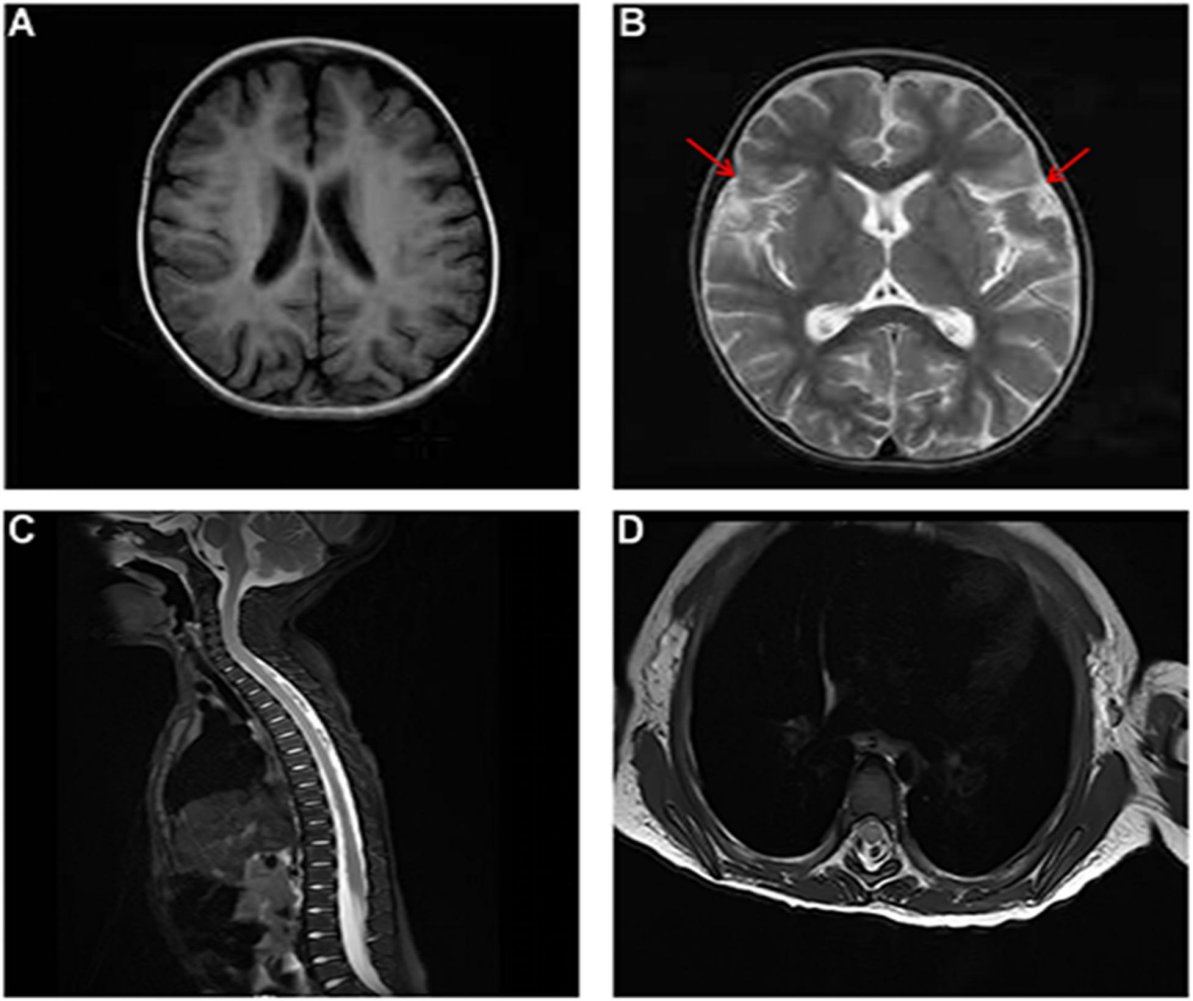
Brain and spinal cord magnetic resonance imaging (MRI) findings. (A,B) Axial brain MRI sequences [(A) T1-weighted; (B) T2-weighted] demonstrate sulcal and cisternal widening (red arrows). (C,D) Sagittal and axial spinal MRI sequences reveal a heterogeneous signal intensity and subtle T2 hyperintensity within the cervicothoracic spinal cord.
